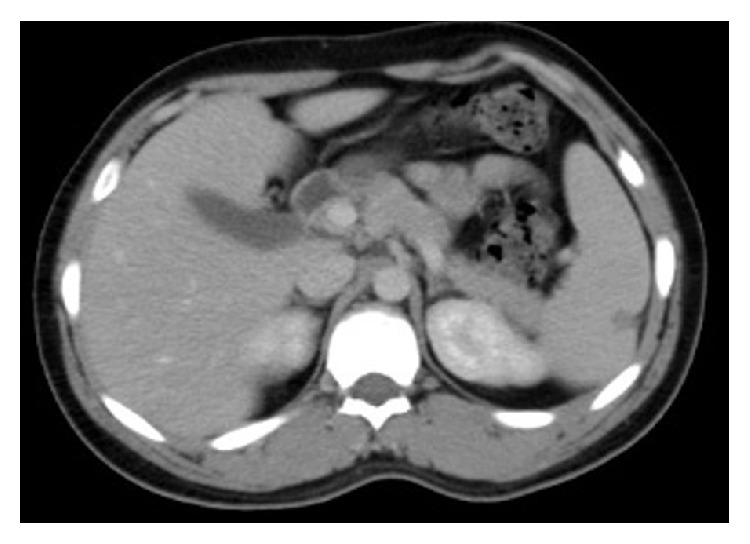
Repeated CT abdomen showing marked regression of the largest lesion while disappearance of others.
